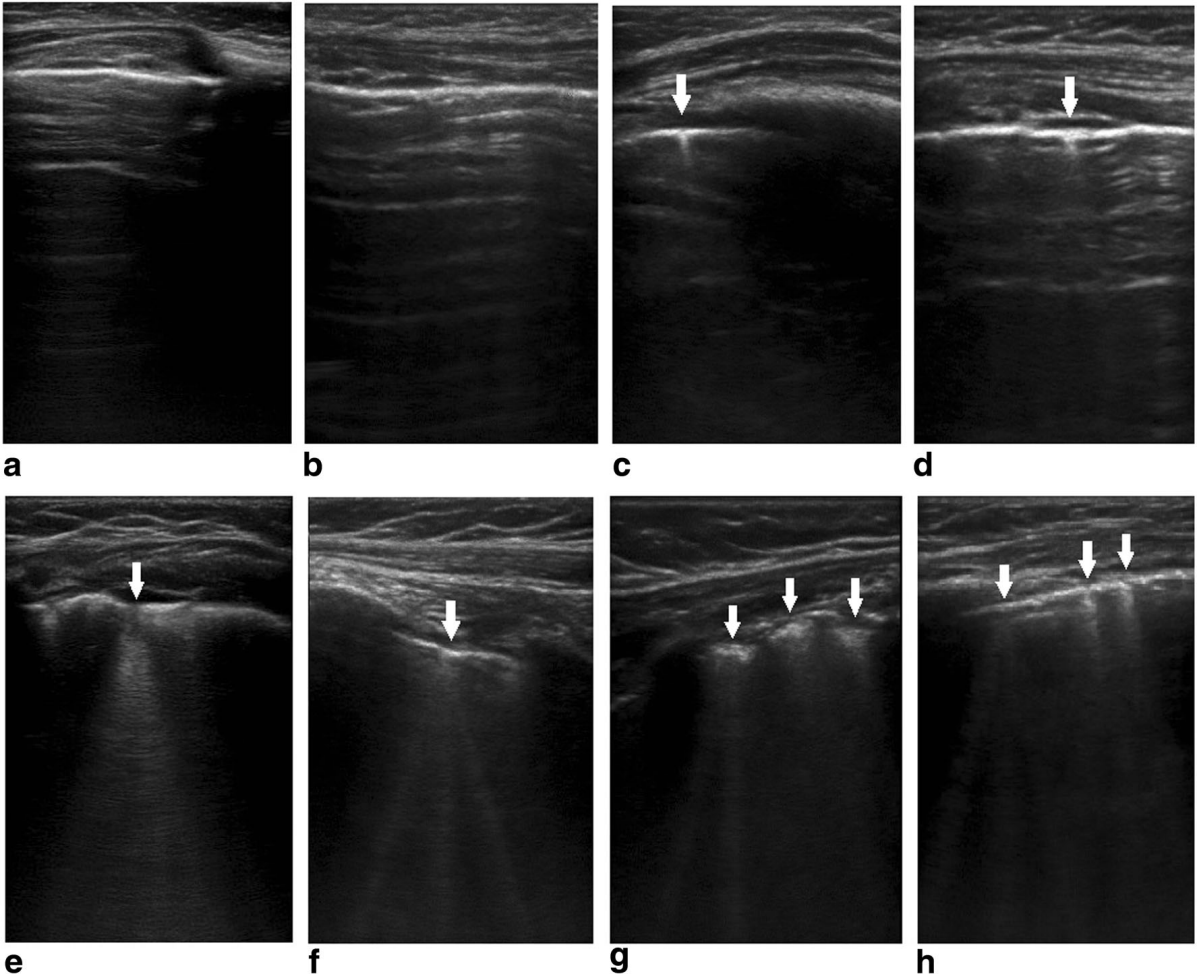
Lung ultrasound B-lines. a, b A-lines (parallel to the pleural line), c, d Z-lines (comet tails that do not obliterate A-lines and do not penetrate to the bottom of the ultrasound window;* arrows*), e, f single B-line (arising from a single point at the pleural line;* arrows*), g, h three B-lines (arising from three distinct points at the pleural line;* arrows*)
